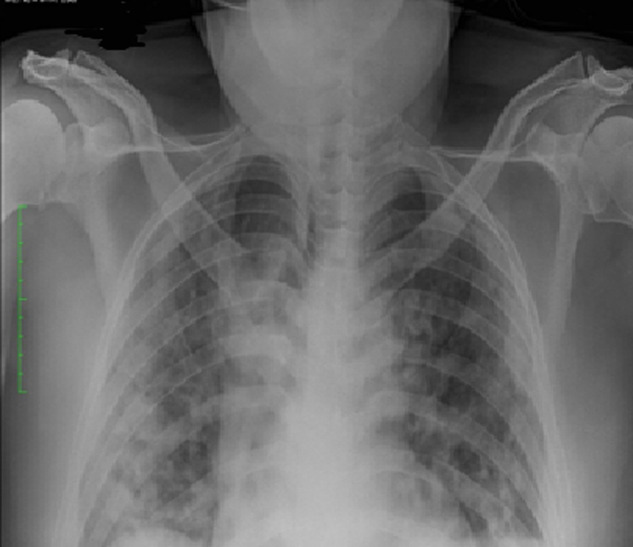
chest radiograph of case 2 on the 5^th^ day of onset of symptoms, showing bilateral nodular opacities with a peripheral and predominantly lower lung zone distribution
